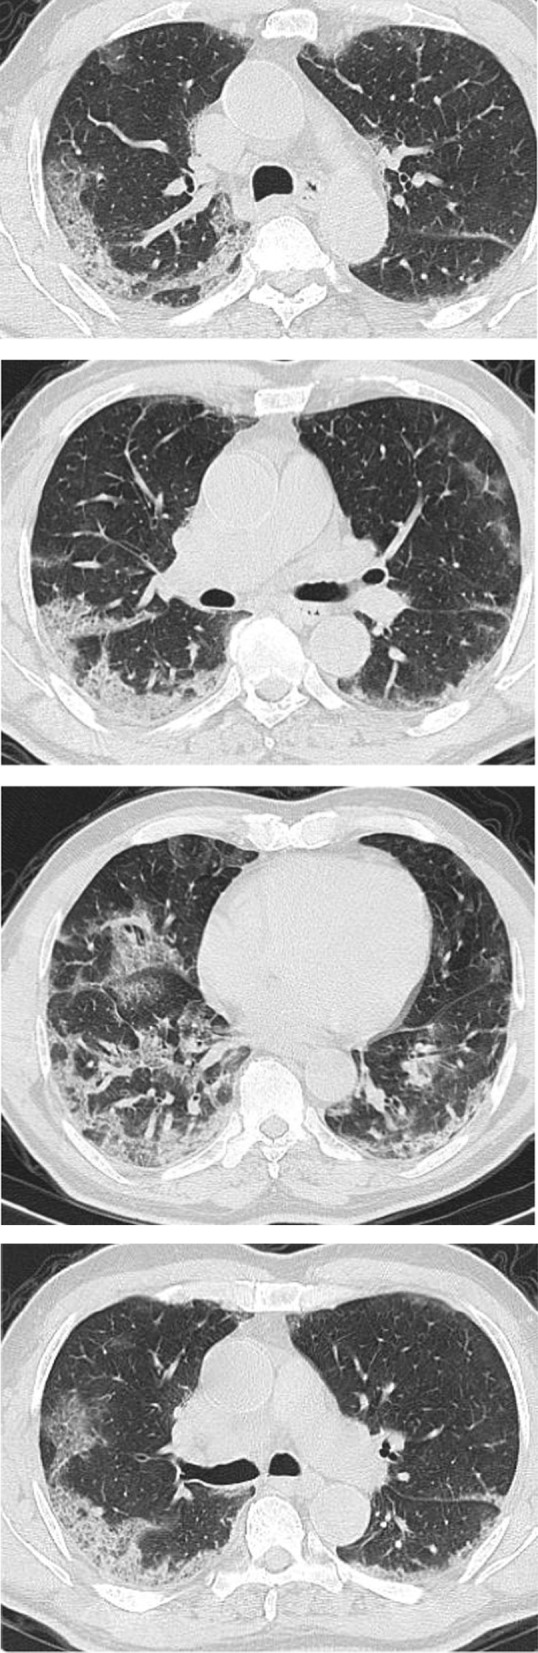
Male patient aged 32 years, with wide areas of consolidation mainly peripheral and subpleural, with crazy pavement appearance. This pattern associated with high CT-SS carried unfavorable prognosis in the current study
